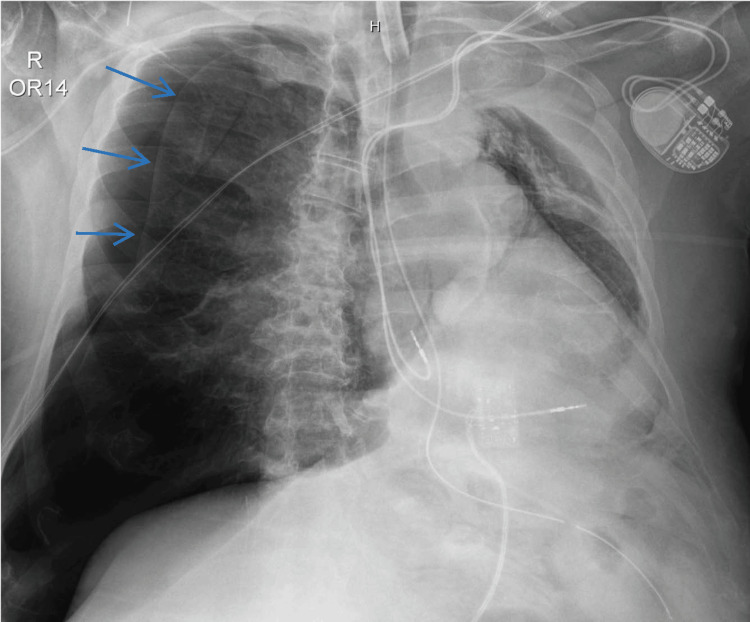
Intraoperative chest X-ray #2 Arrows show right-sided tension pneumothorax, initially read as stable and then subsequently updated to expanding.
